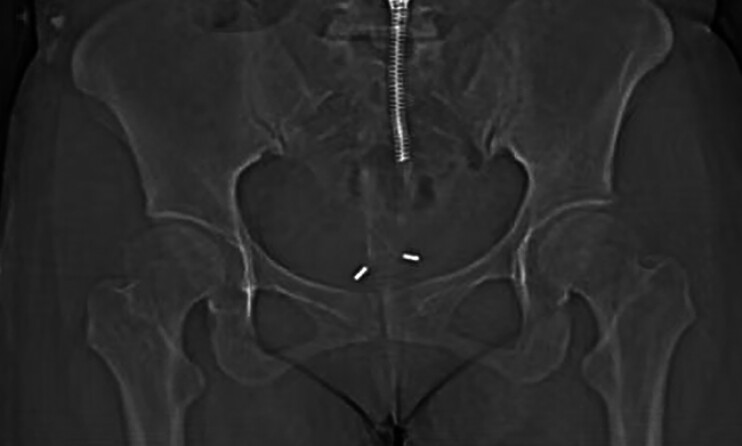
Coronal image of abdominal computed tomography scan indicates an inverted V-shaped metallic shadow in the pelvis.
